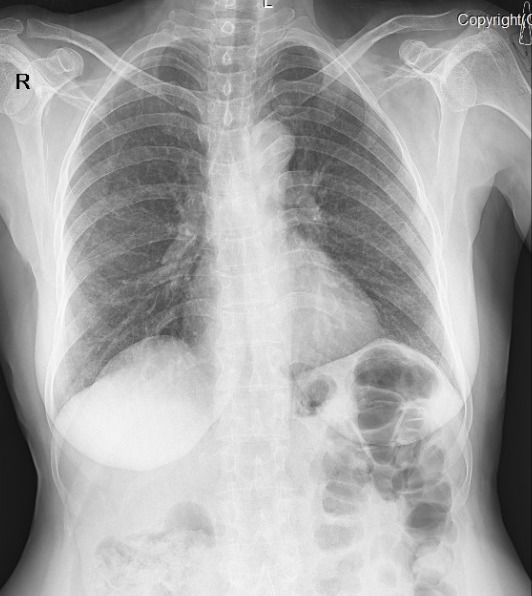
The patient’s chest X-ray
